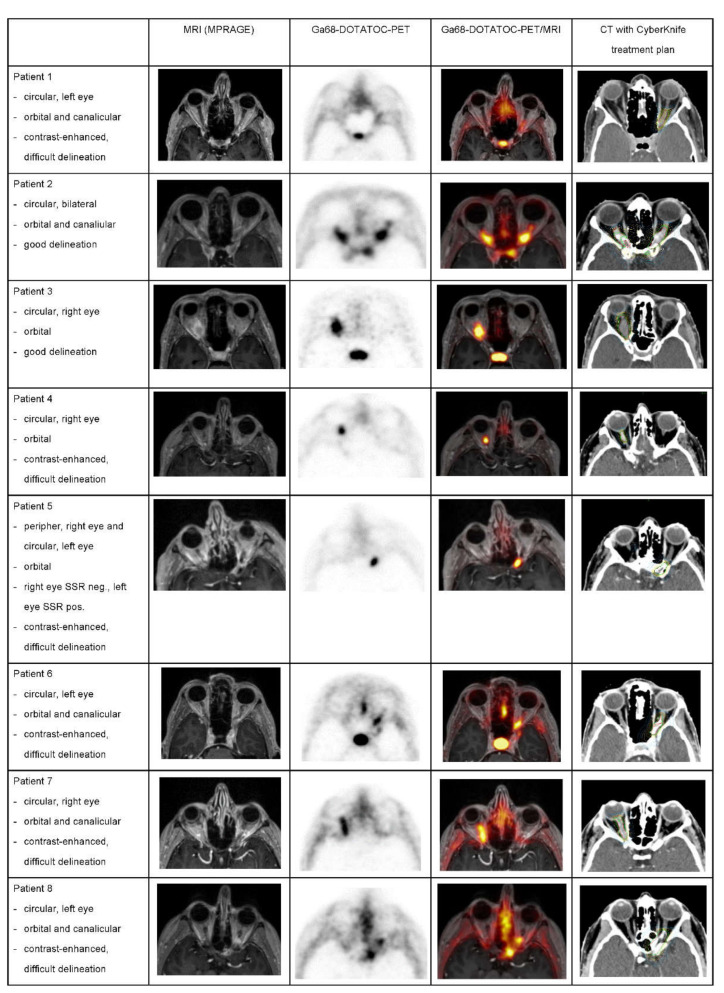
Optic nerve sheath meningiomas (ONSM) on MRI and PET images in all patients and according to the treatment plan. In patients with bilateral ONSM, only one side is presented. MRI, magnetic resonance imaging, PET, positron emission tomography; MPRAGE, contrast-enhanced T1-weighted magnetization-prepared rapid acquisition with gradient echo; Ga68-DOTATOC, gallium-68-labeled (DOTA0-Phe1-Tyr3) octreotide; SSR, somatostatin receptor; Neg, negative; Pos, positive.
